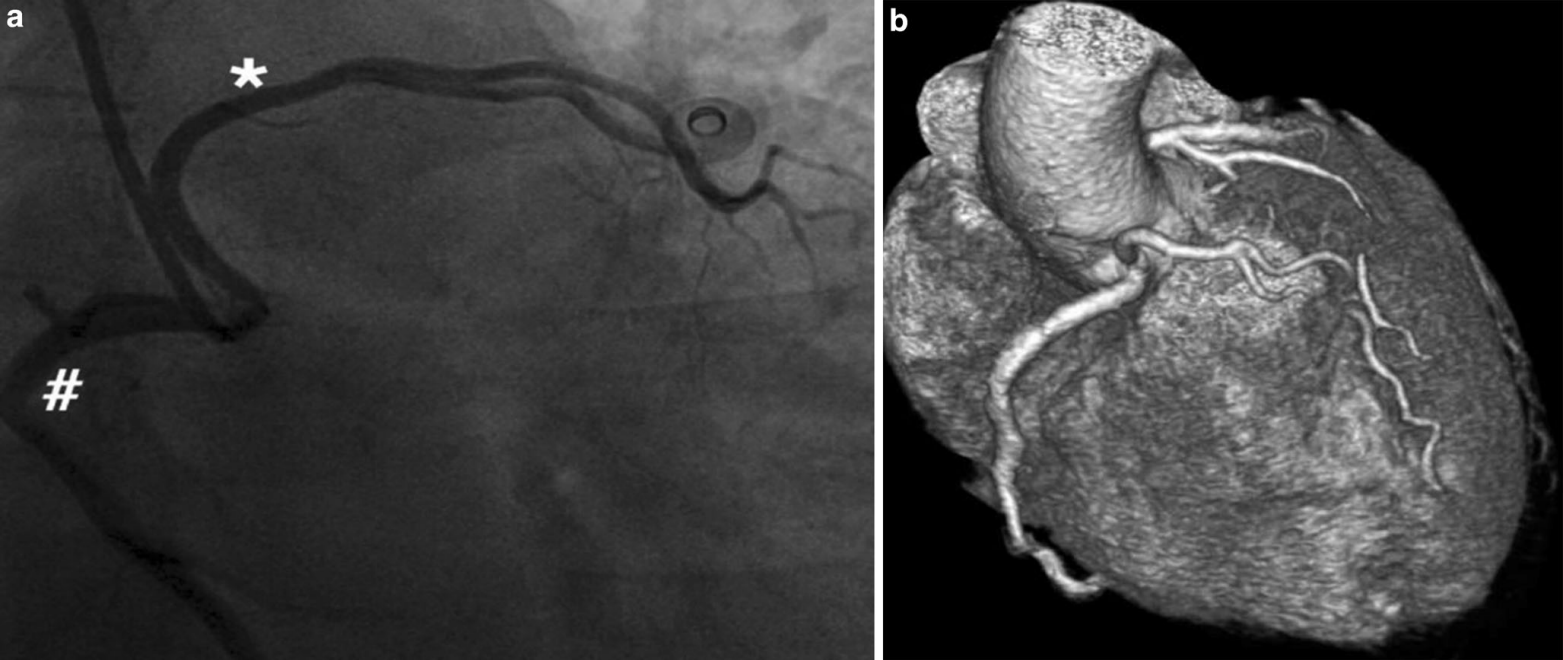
a Coronary angiography displaying opacification of the right coronary cusp of the right coronary artery (#) and the anomalous origin of the left anterior descending coronary artery (*). b Coronary computed tomography revealed no evidence of external compression
